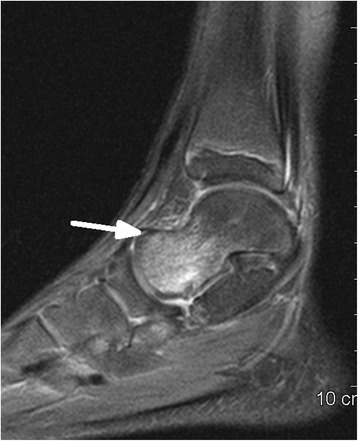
Sagittal PD fat saturated sequence shows marrow oedema of talus (*arrow*) without fracture signs in T1-weighted sequence in Fig. 5
